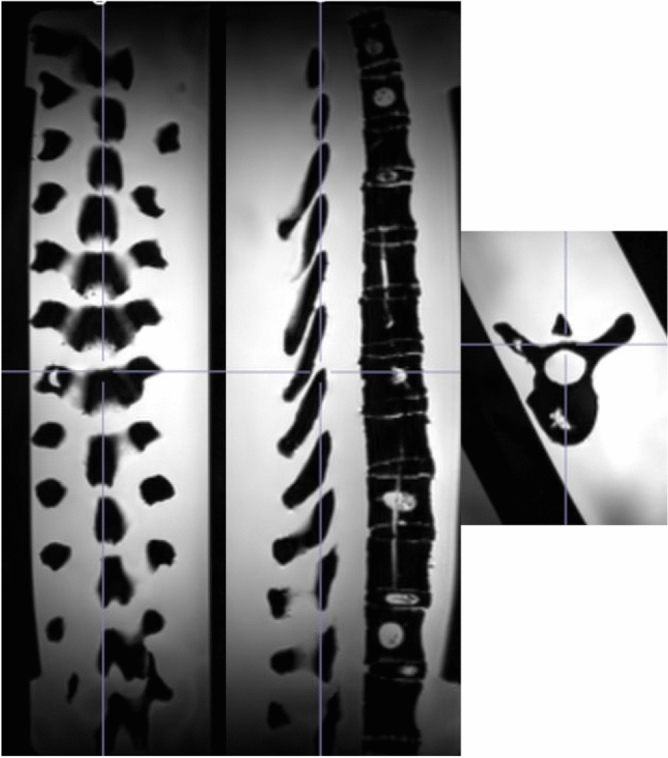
The original MRI volume of the thoracic spine region shown in the posterior coronal, sagittal and transverse planes (left to right).
